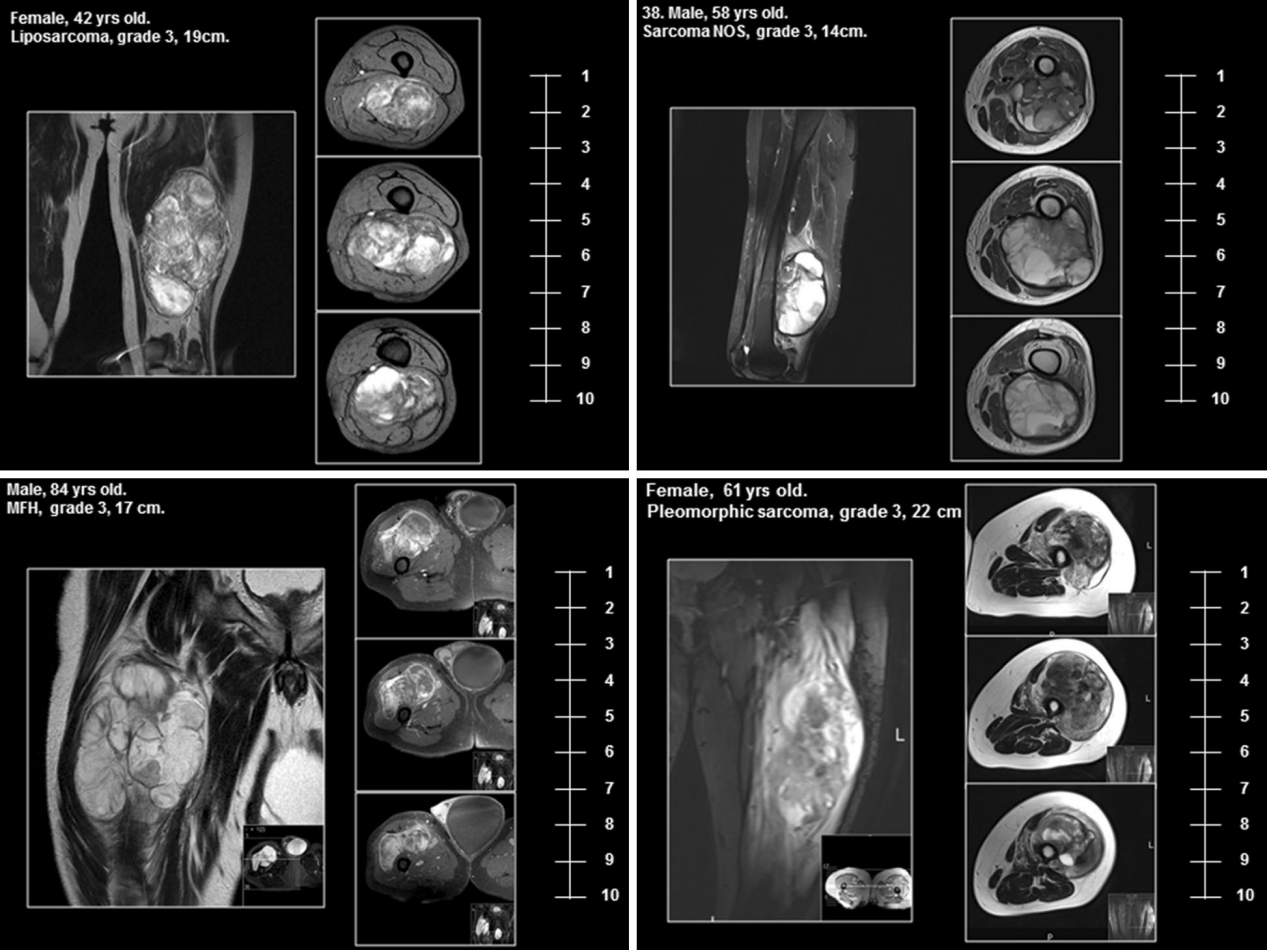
Examples of MRI imaging of patients used for comparison of tumour resectability in cohorts
